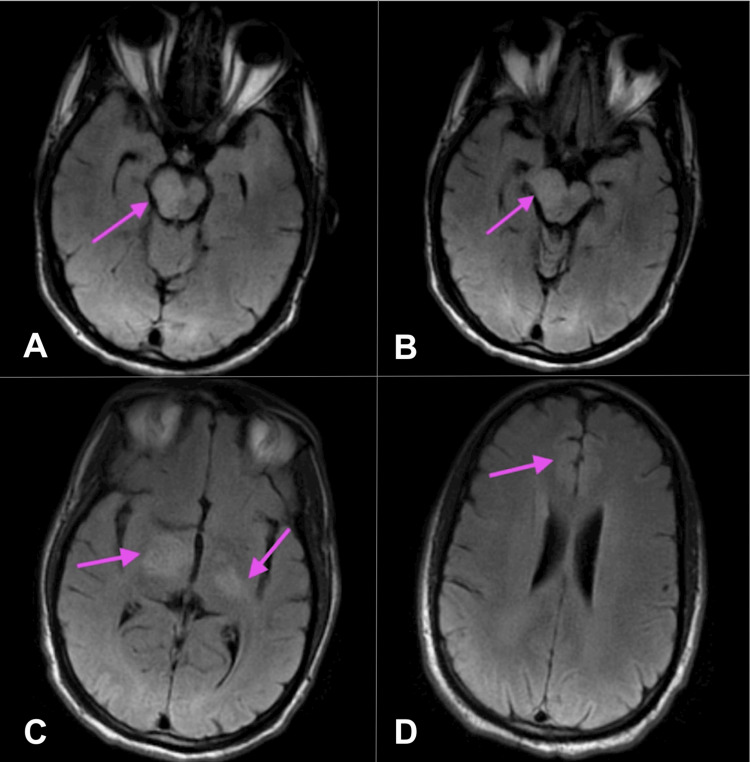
T2 FLAIR MRI brain without contrast (A-D) Differing axial view depths of the brain. MRI brain without contrast (T2 FLAIR) showing hyperintense area extending from the mid-right pons through the midbrain, thalamus, and into the posterior limb of the right internal capsule, concerning for demyelination. Arrows highlight the areas of concern. FLAIR: fluid-attenuated inversion recovery; MRI: magnetic resonance imaging
